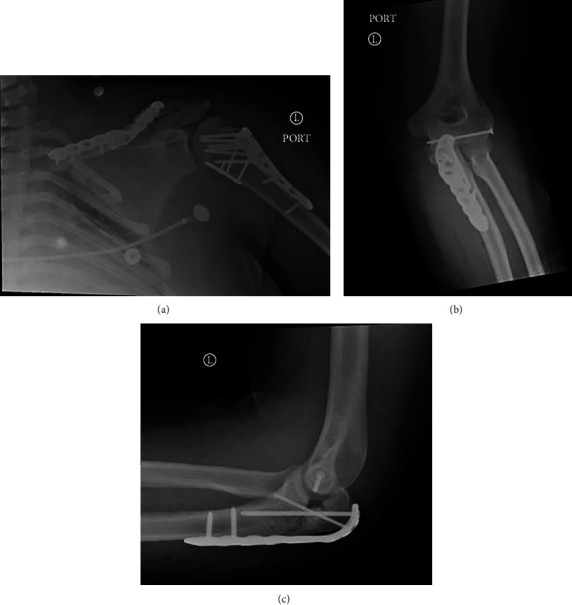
(a) Postoperative anteroposterior X-ray of the left shoulder demonstrating plate and screw fixation of the left clavicle and proximal humerus. (b) Anteroposterior and (c) lateral X-ray of the left elbow demonstrating plate and screw fixation of the proximal humerus and olecranon process.
